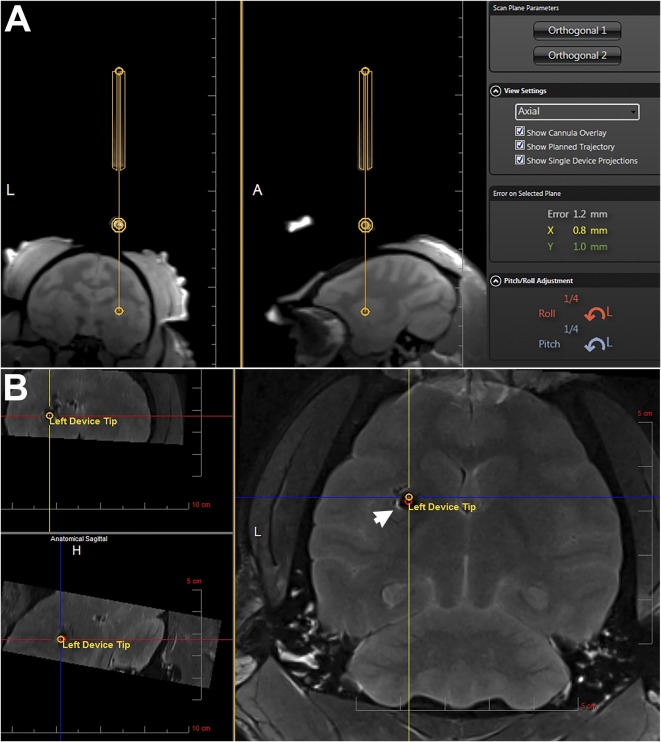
Finalizing trajectory and cannula entry. (A): Representative slices of the orthogonal alignment images shown in the ClearPoint software. The yellow lines outline the segmentation of the cannula guide. The software‐calculated adjustments are shown in orange (Roll) and blue (Pitch) on the bottom right. (B): Postinjection magnetic resonance image overlaid with the planned target and cannula tip (Left Device Tip) as calculated by the ClearPoint software showing the superparamagnetic iron oxide‐labeled neural stem cell graft (arrow, hypointense area) on target. Abbreviations: A, anterior; H, head; L, left.
